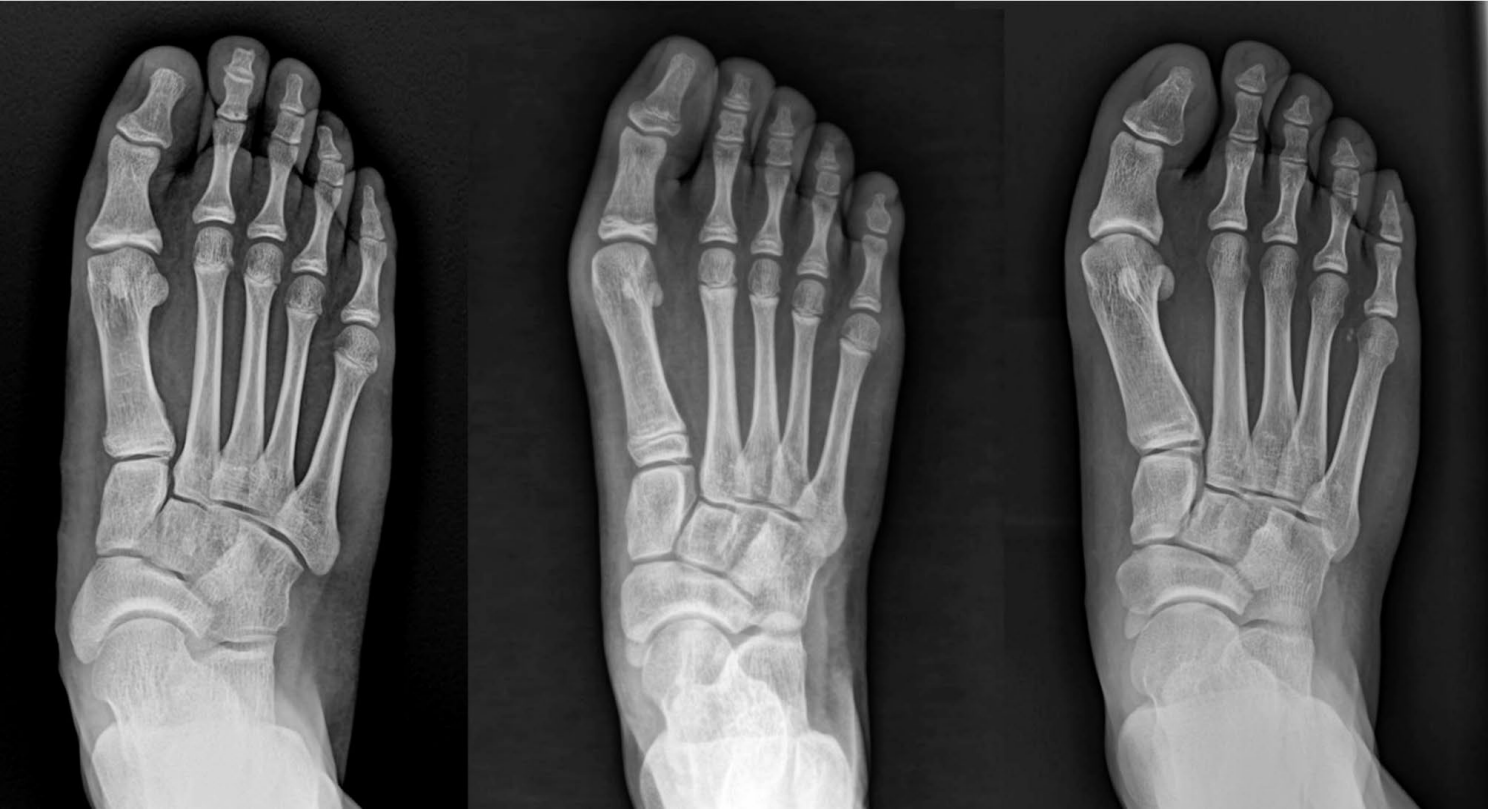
Standing anteroposterior foot radiographs of the patients with juvenile-onset hallux valgus with concomitant hallux valgus interphalangeus
